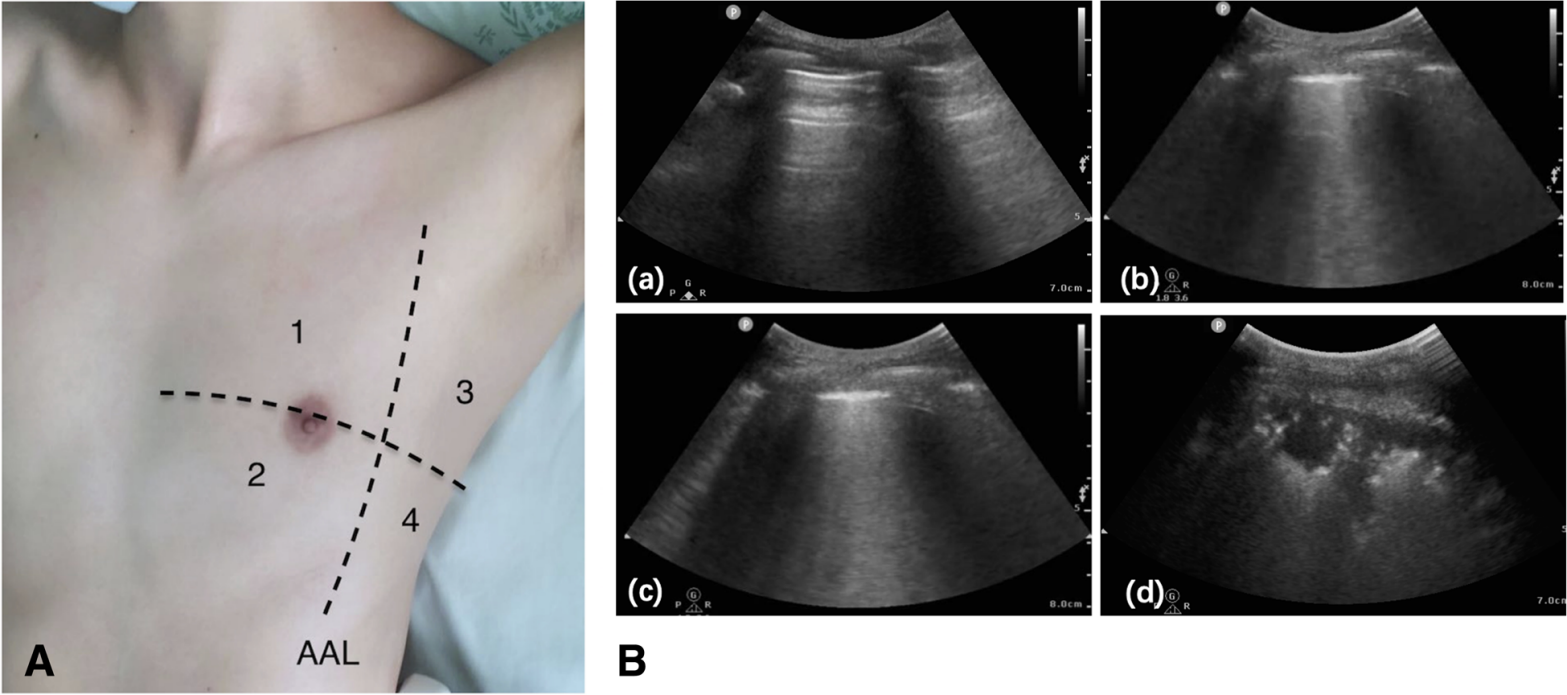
Eight-zone lung ultrasound examination protocol and lung ultrasound pattern. A: Each hemithorax is separated into four quadrants: anterior and lateral zones (separated by the anterior axillary lines) with each one divided into upper and lower portions. AAL indicates the anterior axillary line. B: Lung ultrasound pattern. (a): A pattern; (b): B1 pattern; (c): B2 pattern; (d): C pattern (lung consolidation).
